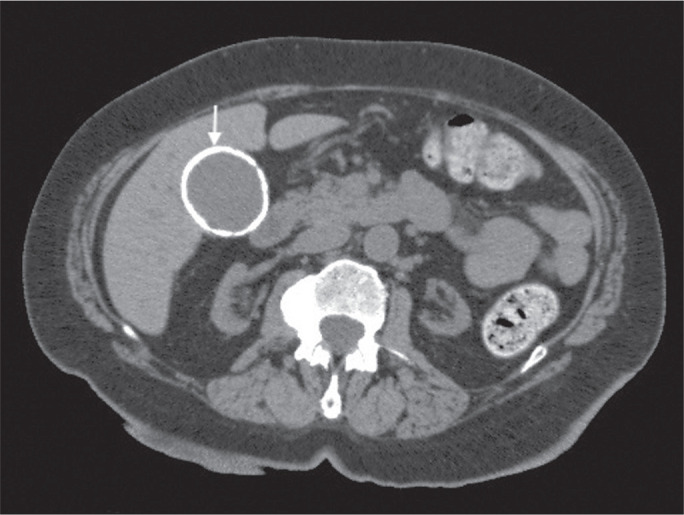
Chest CT of a 66-year-old female with porcelain gallbladder
